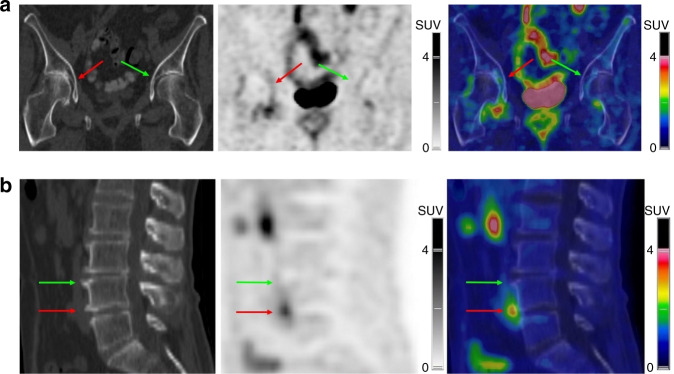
Location of ^18^F-FPRGD2 uptake. ^18^F-FPRGD2 PET/CT images (A: CT; B: PET; C: fused PET/CT) from an 80-year-old patient with bilateral coxofemoral osteoarthritis. ^18^F-FPRGD2 uptake is higher in the right joint, including the region of articular cartilage identified by the red arrows (SUVmax 2.3 target SUVmax-to-muscle SUVmax ratio 3.1). Based on CT images, degenerative changes are also more advanced in the right coxofemoral joint (a). ^18^F-FPRGD_2_ PET/CT images (A: CT; B: PET; C: fused PET/CT) of a 74-year-old patient showing osteophytes at the anterior margins of the L3–L4 disc (pointed to by green arrows) and L4–L5 disc (pointed to by red arrows), yet only the latter showed high ^18^F-FPRGD_2_ uptake (maximum standardized uptake value SUV_max_ 3.2; target SUV_max_-to-muscle SUV_max_ ratio 6.5) (b)
